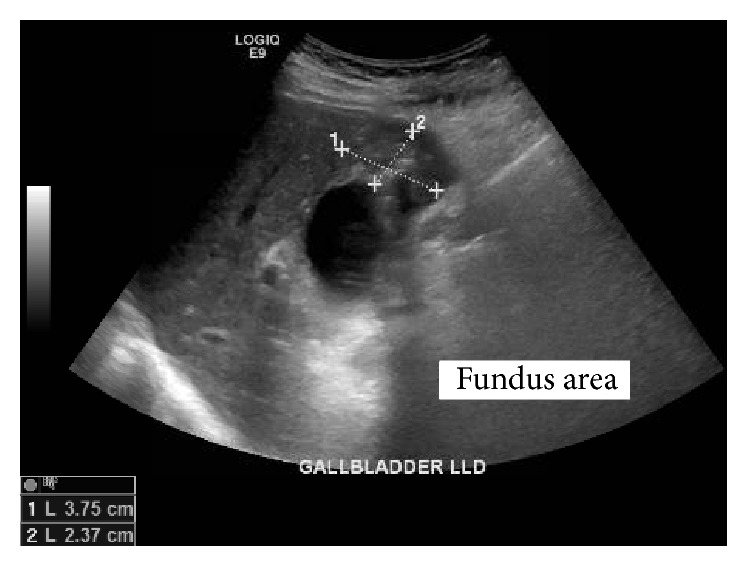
Right upper quadrant ultrasound showing 3.8 × 2.4 × 3.5 heterogeneous mass with small calcification emanating from the gallbladder fundus.
